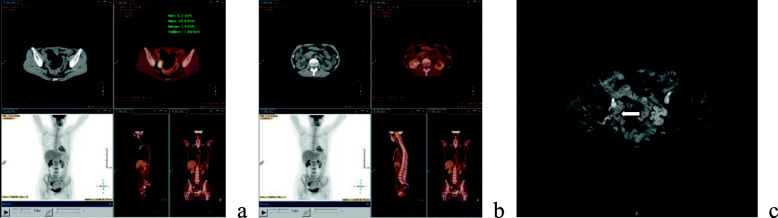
Recurrent lesion: a PET-CT lesion recurred from ovarian cancer at right pelvic cavity. b Mild hydronephrosis. c MRI lesion recurred from ovarian cancer after four cycles of chemotherapy
